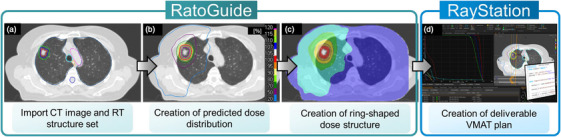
The outline of automated planning procedure using RatoGuide and RayStation. (a) DICOM data of planning CT and RT structure set were imported to RatoGuide software. (b) Dose distribution was predicted using RatoGuide. (c) RatoGuide automatically generated dose structures for optimization based on predicted dose. CT images and RT structure set were exported with dose structures to RayStation. (d) Automated treatment planning on RayStation using scripts. The calculated dose distributions were evaluated. CT, computed tomography; RT, radiation therapy.
